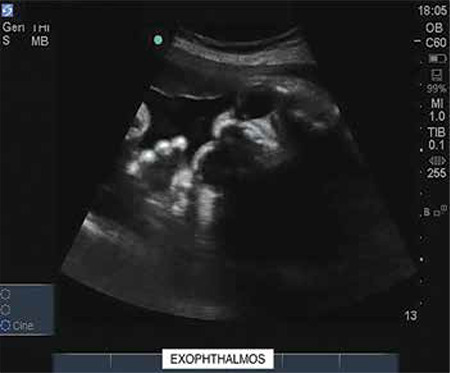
Ultrasound image (2-dimensional) of transverse section of fetal face demonstrating bulging eyeballs, suggestive of orbital proptosis
